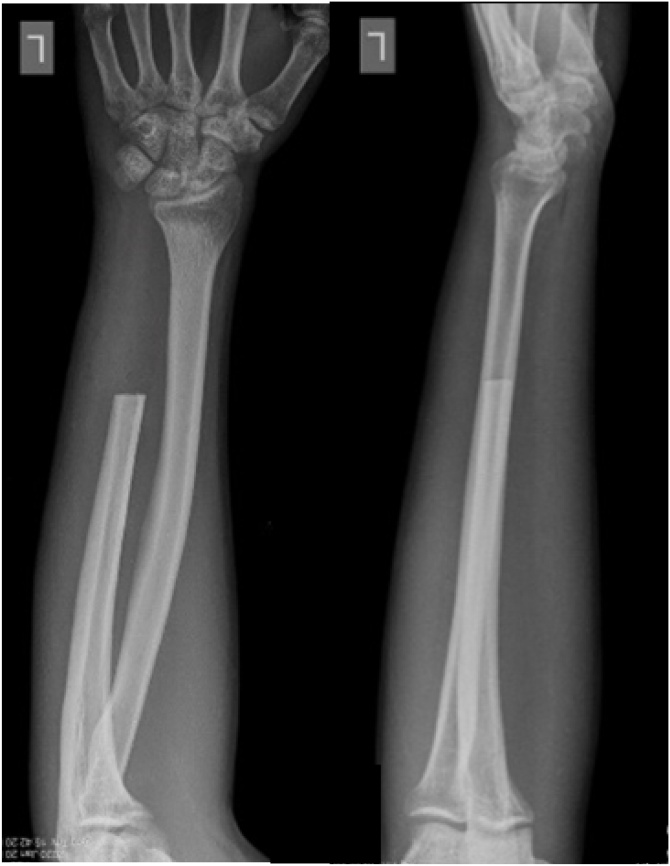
Postoperative radiograph after 3 weeks.
